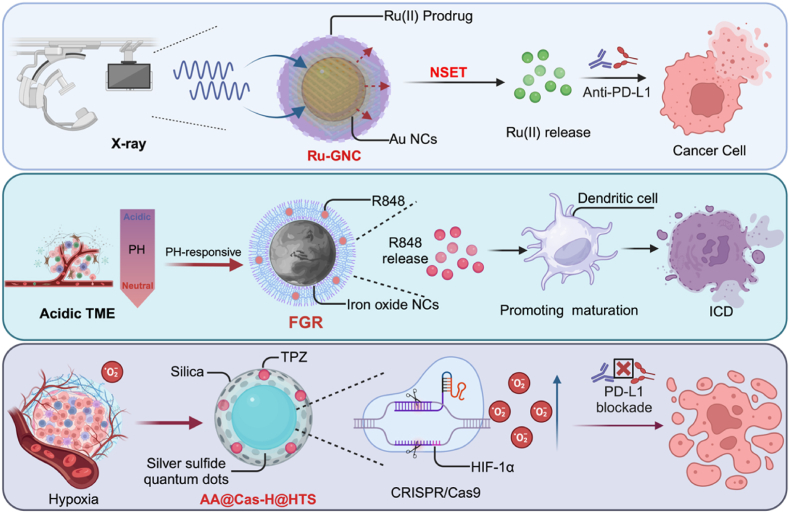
Inorganic NC-based cancer therapy strategies. Ru(II) Prodrug: X-ray activates Ru-GNC via NSET, releasing Ru(II). Combined with Anti-PD-L1, induces ICD in cancer cells.Acidic TME: pH-responsive FGR (Fe^3+^/R848 NCs) releases R848 in acidic TME, promoting DC maturation and ICD.Hypoxia: Ag_2_S QDs deliver AA@Cas-H@HTS (CRISPR/Cas9) to inhibit HIF-1α/PD-L1, alleviating hypoxia and enhancing T-cell immunity.
